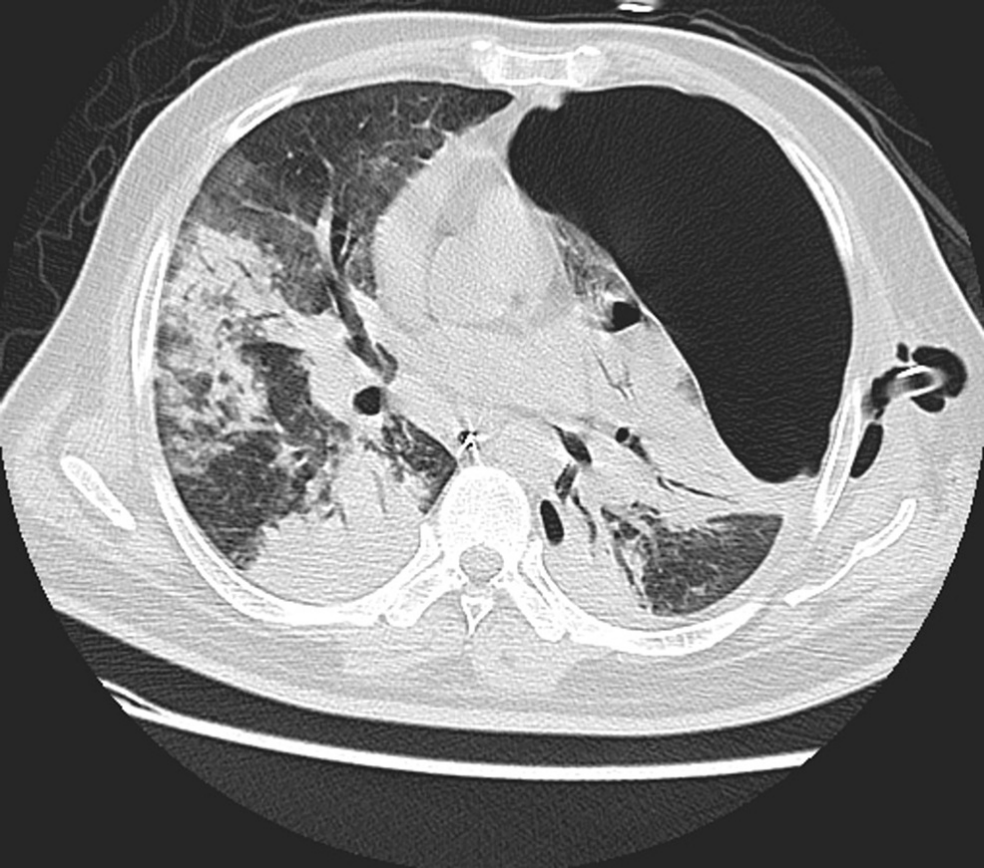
Selected axial CT image showing extensive bilateral consolidation and large left pneumothorax
